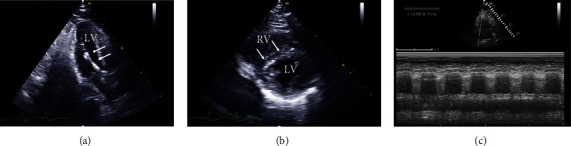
(a) Transthoracic echocardiogram (TTE) 3-chamber showing the Impella CP percutaneous left ventricular assist device (arrows) within the left ventricle (LV). (b) TTE short axis view demonstrating septal flattening (arrows) indicating right ventricular (RV) pressure/volume overload; the RV is dilated as well. (c) TTE M-mode of the tricuspid annulus showing decreased (dashed line) tricuspid annular plane systolic excursion (TAPSE) of 0.73 cm suggesting RV dysfunction.
